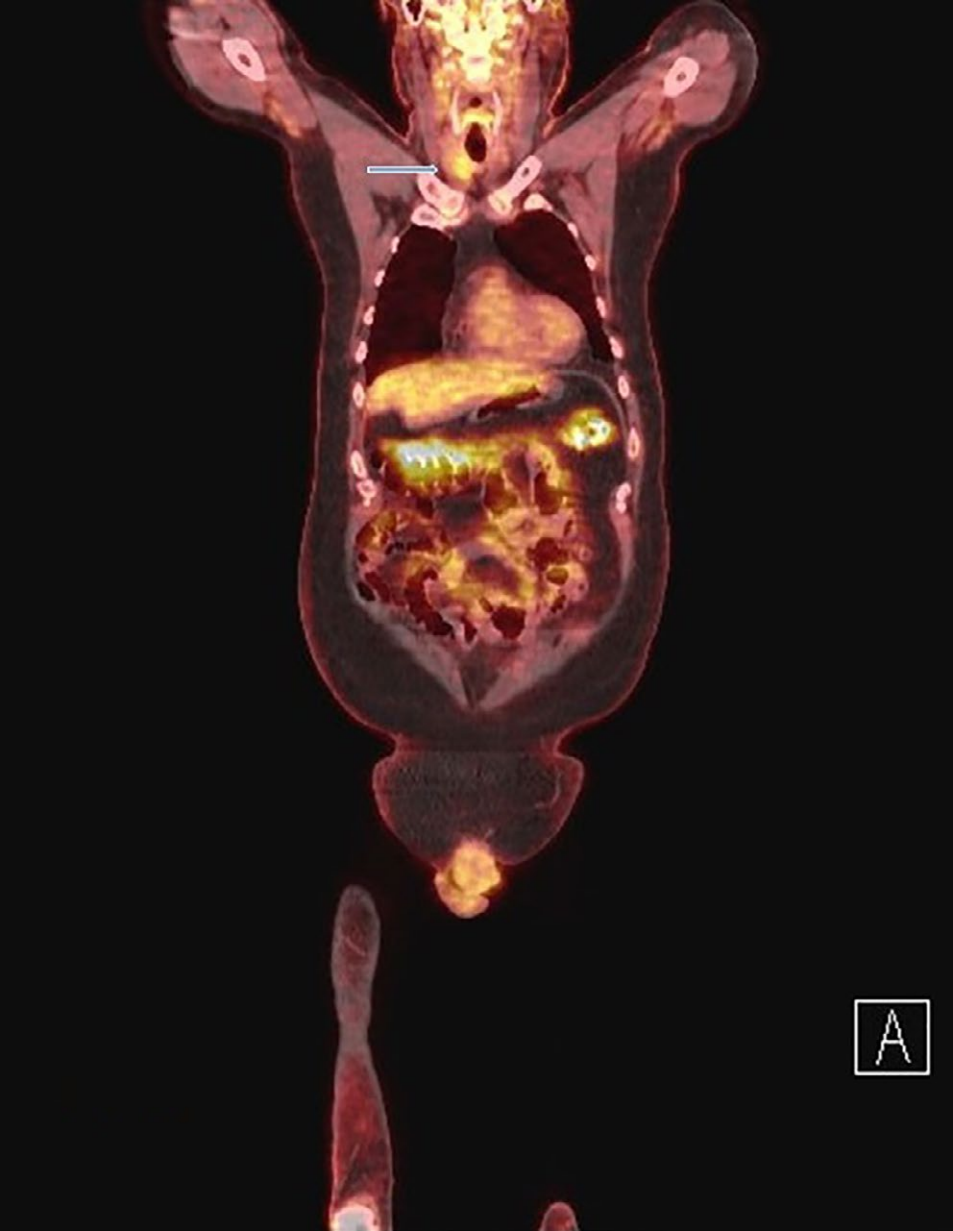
PET‐CT demonstrating an FDG‐avid right‐sided thyroid nodule with a standard uptake value of 4.58
